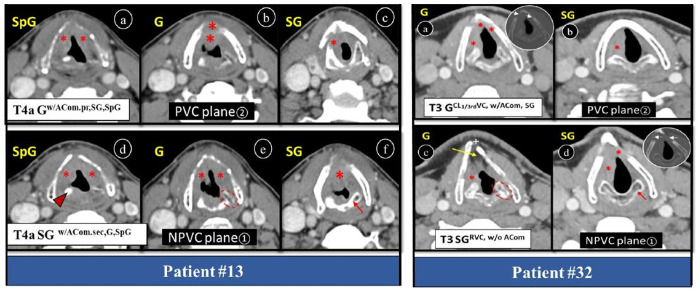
Axial post-contrast CT larynx showing change in origin (considering tumor bulk) and extension of T4a stage glottic tumor due to CT plane. Patient#13: Plane② (a-c) showing ACom origin glottic tumor, while plane① (d-f) SG origin. Asterisks (*) showing areas of tumor extension (SpG, G, ACom, SG, TC), arrowhead is upper part of AC at SpG level, dotted oval is CAJ at glottic level, arrow is SG cricoid cartilage. Patient#32: Plane② (a, b) showing right glottic tumor extending to ACom, CL < 1/3rd of VC and SG, while plane① (c, d) showing right SG tumor extending to ipsilateral G without ACom and CL VC extension. Asterisks (*) showing areas of tumor extension (G, CL G, ACom, SG), dotted oval is CAJ at glottic level, short arrow is SG cricoid cartilage. Also note false anatomical landmarks of G in NPVC plane, i.e., fat (yellow long arrow) and open thyroid notch (+). *SpG = supraglottis, G = glottis, SG = subglottis, ACom = anterior commissure, w/ACom.pr=with primary origin from anterior commissure, w/ACom.sec=with secondary invasion of anterior commissure, NPVC = non-parallel vocal cord, PVC = parallel vocal cord, AC = arytenoid cartilage, CAJ = cricoarytenoid joint, w/,w/o=with and without, RVC = right vocal cord, CL = contralateral.*
